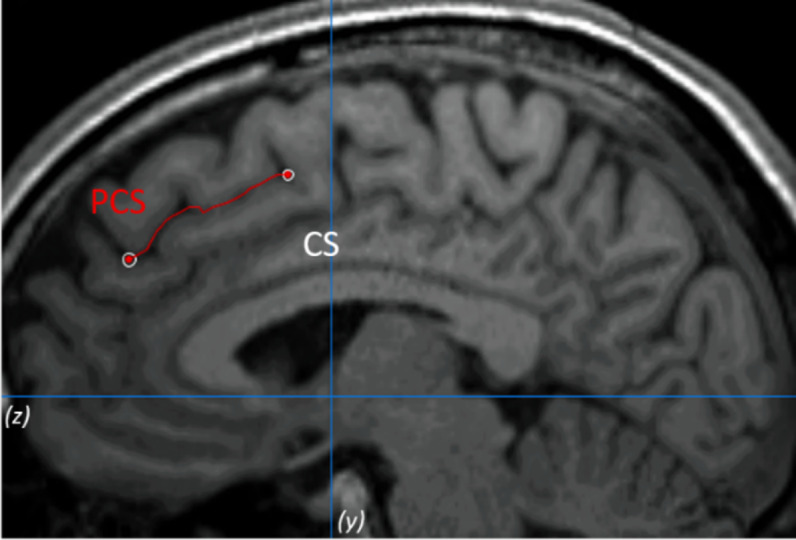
Example of the paracingulate sulcus (PCS) measurement on anatomical magnetic resonance imaging. The PCS is marked in red line and lies dorsal and parallel to the cingulate sulcus. Measurement was performed within the first quadrant (defined by *z* < 0 and *y* > 0) and on the fourth sagittal slice for both hemispheres.
